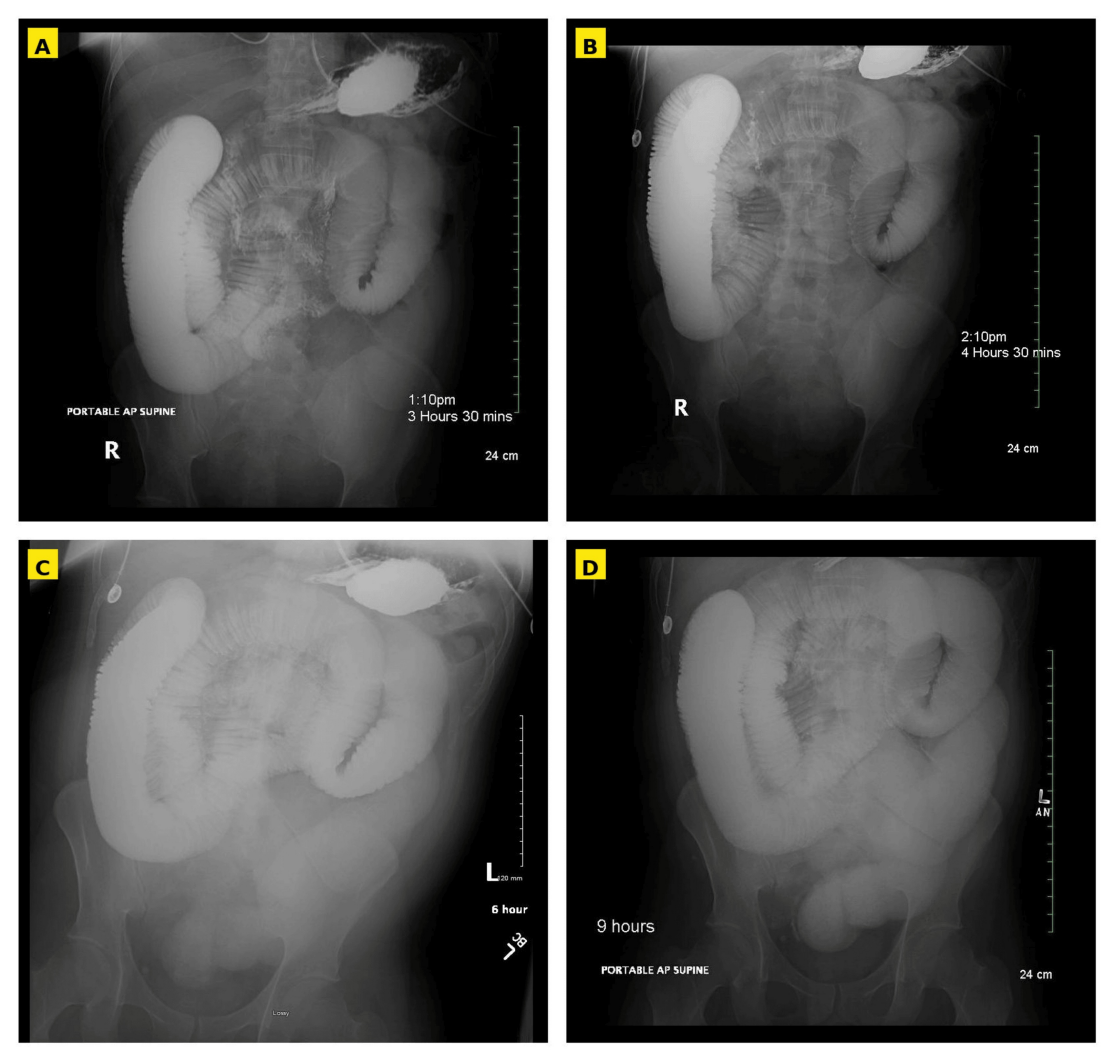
Fluoroscopic Small-Bowel Follow-Through. Abdominal radiographs obtained at A. 3.5 hours, B. 4.5 hours, C. 6 hours, and D. 9 hours after administration of fluoroscopic contrast. At 3.5 hours, contrast remained within the dilated small bowel without progression into the colon, unchanged at the 6-hour film, and no further progression was seen between the 6-hour and 9-hour film.
